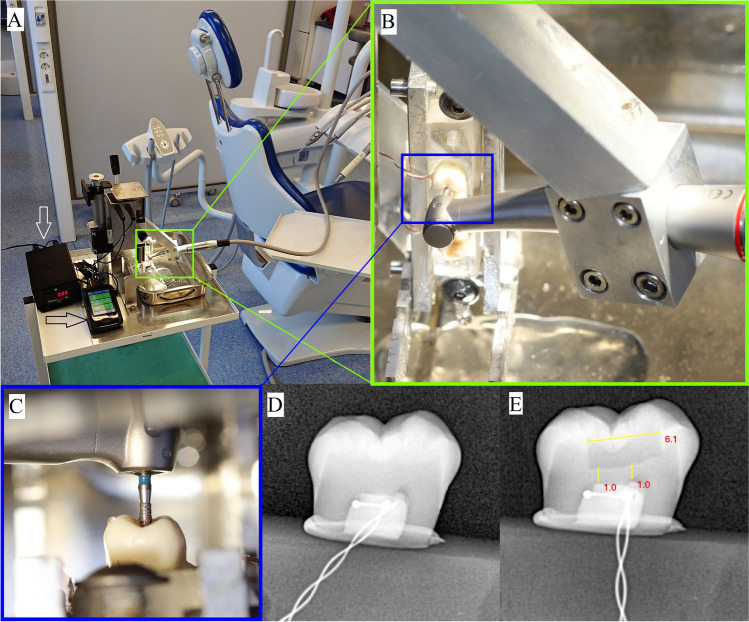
The drilling tower and restorative cavity drilling A the drilling tower was attached to a regular dental clinical unit. The time measurement unit (white arrow) and temperature registration device (black arrow) cooperated with the drilling tower; B two molars were attached to each acrylic plate; C the drill’s initial position in the hole in the tooth (as the starting point of cavity preparation); D before drillings, the placement and position of the thermosensor was controlled with intraoral radiographs; E after cavity preparations, the preoperative caliper measurement method was regularly controlled. It is clear that drilling with inclination was possible with the adjustment of the acrylic plate holding the metal frame in the tower
